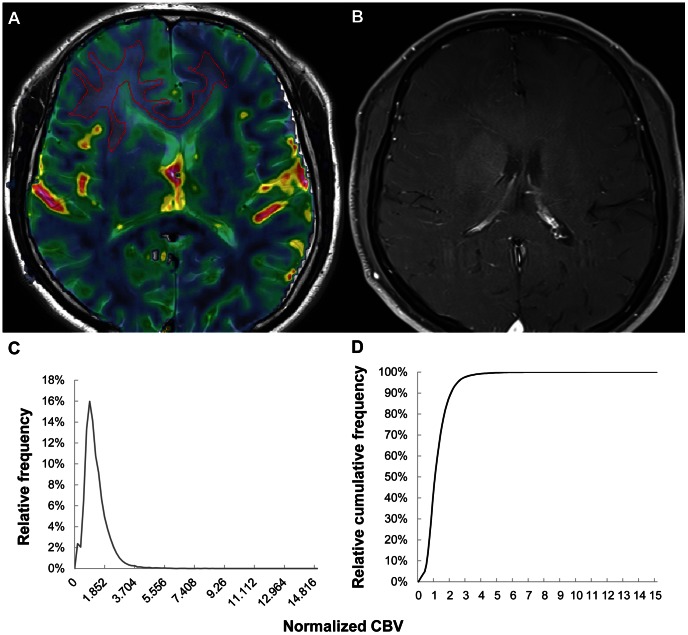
Images of a 55-year-old male with a grade II astrocytoma. (A) ROI-drawn rCBV map overlaid on a T2-weighted image, (B) contrast-enhanced T1-weighted image, (C) histogram of nCBV and (D) cumulative nCBV histogram. The 99^th^ percentile value of the cumulative nCBV histogram was 3.703. ROI = region of interest; rCBV = relative CBV; nCBV = normalized CBV.
